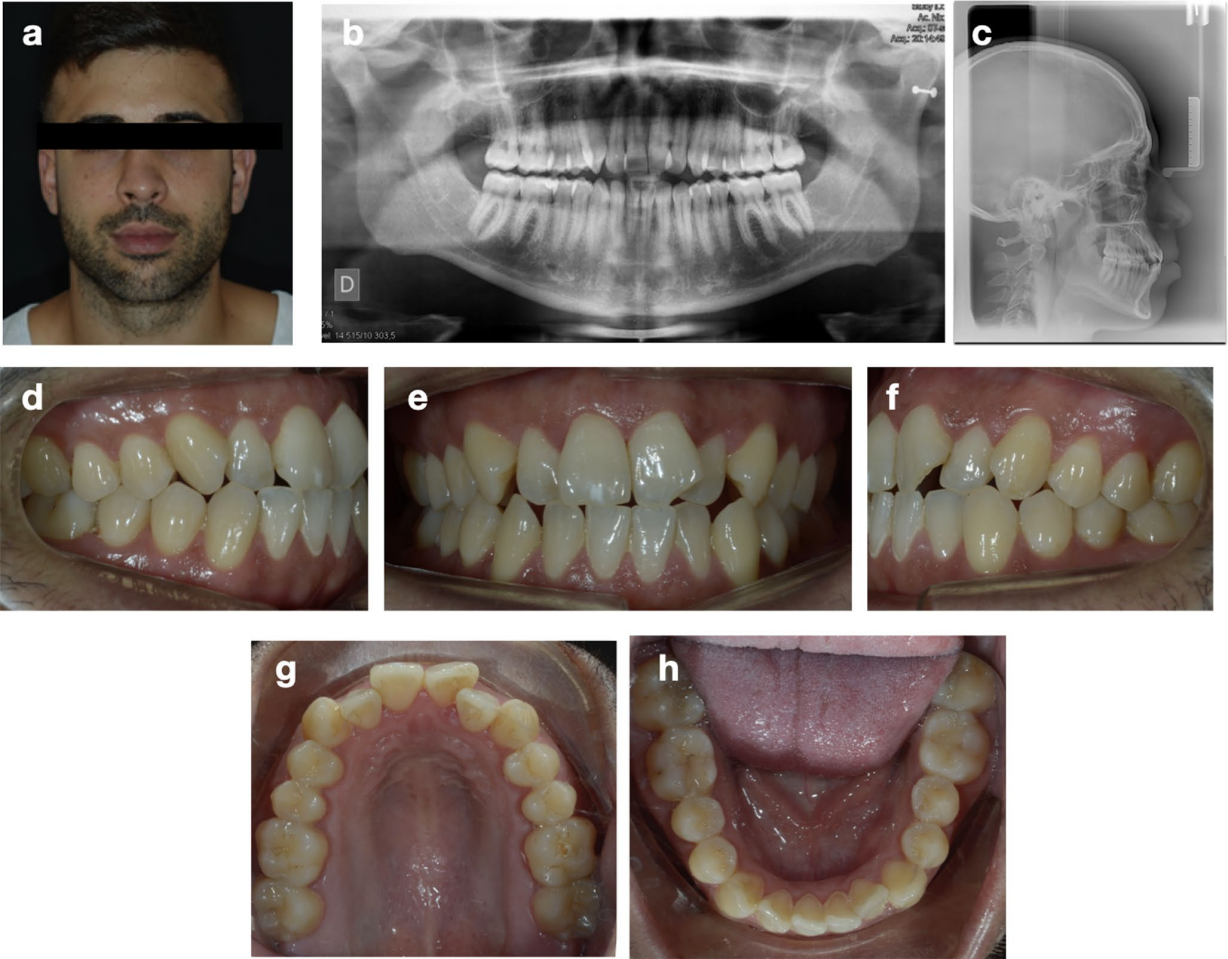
Case presentation. Pretreatment facial photograph (a), panoramic (b) and lateral cephalometric radiographs (c), and intraoral photographs (d-h)
